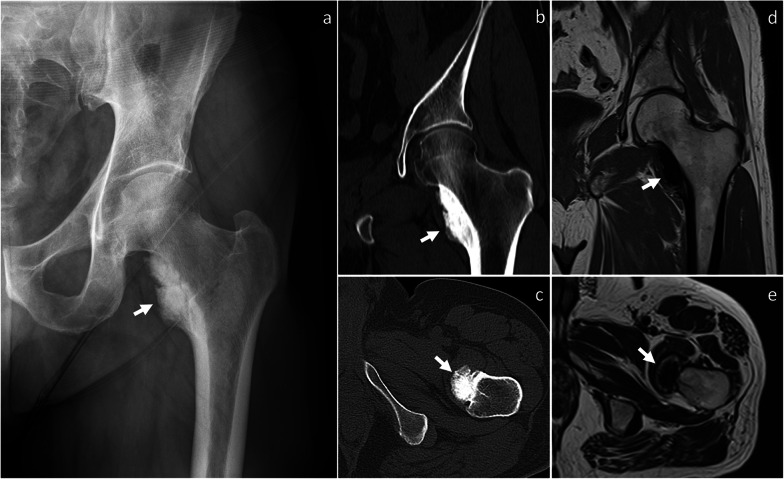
Parosteal osteosarcoma. On X-rays (a), an exophytic heavily ossified mass is seen in contiguity with the medial aspect of the femoral neck. On coronal (b) and axial (c) CT images, the mass is denser centrally than at the periphery. The mass shows predominantly low signal representing mineralized component on both coronal T1-weighted (d) and axial T2-weighted (e) MRI sequences. Parosteal osteosarcoma was pathologically proven. Arrow points at the lesion in all images
